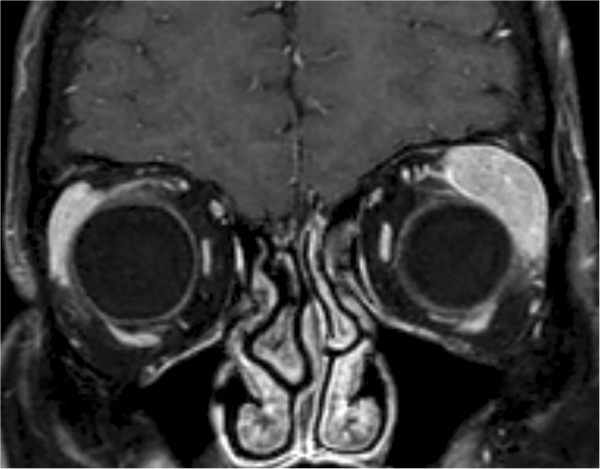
MRI of orbital tumor.
